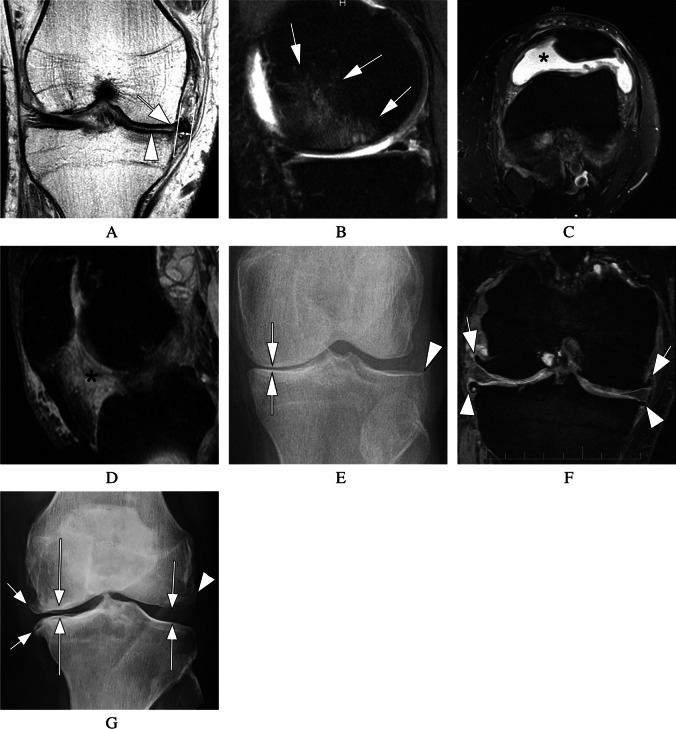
Phenotypic characterization. A The cartilage/meniscus phenotype is characterized by severe meniscal damage depicted in this example as partial meniscal maceration of the medial meniscal body (arrow) and is commonly associated with severe cartilage loss (arrows point to diffuse superficial cartilage damage of the medial tibia). There is severe meniscal extrusion (parallel lines and double-headed small arrow). In addition, there is diffuse superficial cartilage damage at the medial femur. Different definitions of a cartilage-meniscus phenotype have been proposed depending on the amount of cartilage damage and meniscal involvement (Roemer FW et al., Patterns of progression differ between Kellgren-Lawrence 2 and 3 knees fulfilling different definitions of a cartilage-meniscus phenotype in the Foundation for National Institutes of Health Osteoarthritis Biomarkers study (FNIH). Osteoarthritis and Cartilage Open 2022;4(3): 100284.). Depending on the definition, this knee may also fulfill the imaging requirements of an atrophic phenotype, characterized by advanced cartilage loss and little or no marginal osteophytes. B Bone phenotype. A large bone marrow lesion (BML) is present in the medial central subregion of the medial femur (grade 3, arrows). The size of the BML defines this knee as a bone phenotype. C The inflammatory phenotype is characterized by severe joint effusion-synovitis (asterisk). D So-called Hoffa-synovitis, a non-specific surrogate of whole knee synovitis, is another manifestation of inflammation and is considered for the classification of an inflammatory structural phenotype. E The atrophic phenotype is characterized by severe cartilage loss without relevant osteophyte formation. It can be diagnosed by radiography. Anterior–posterior radiograph shows severe medial joint space narrowing (arrows), defining this knee as Kellgren-Lawrence grade 3. There is, however, only a tiny, equivocal osteophyte at the lateral tibia (arrow). The discrepancy between joint space narrowing (a surrogate for cartilage and meniscal damage, and meniscal extrusion) defines this knee as having an atrophic phenotype. F The hypertrophic phenotype is characterized by large osteophytes with only minor cartilage loss and can be defined either radiographically based on joint space narrowing or by MRI based on cartilage integrity or damage. Coronal dual echo at steady state (DESS) reformatted image shows large osteophytes at the medial and lateral femoral joint margin (arrows) and moderate-sized osteophytes at the medial and lateral tibia (arrowheads). G Example of the hypertrophic phenotype as visualized by X-ray. Large marginal osteophytes are seen at the medial compartment (short arrows) but also laterally (arrowhead). The medial and lateral joint space is largely preserved (long arrows)
